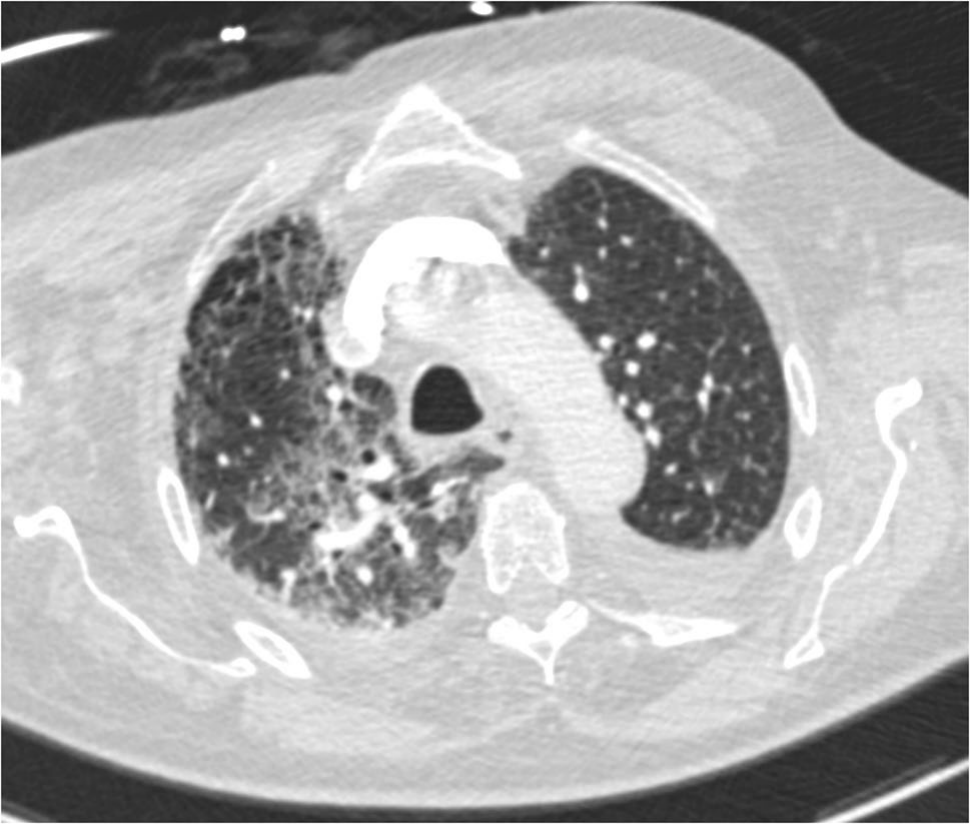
Chest computed tomography of the SARS-CoV-2-positive 64-year-old male patient with right-sided multilobe involvement and ground-glass opacity and left-sided pleural effusion
